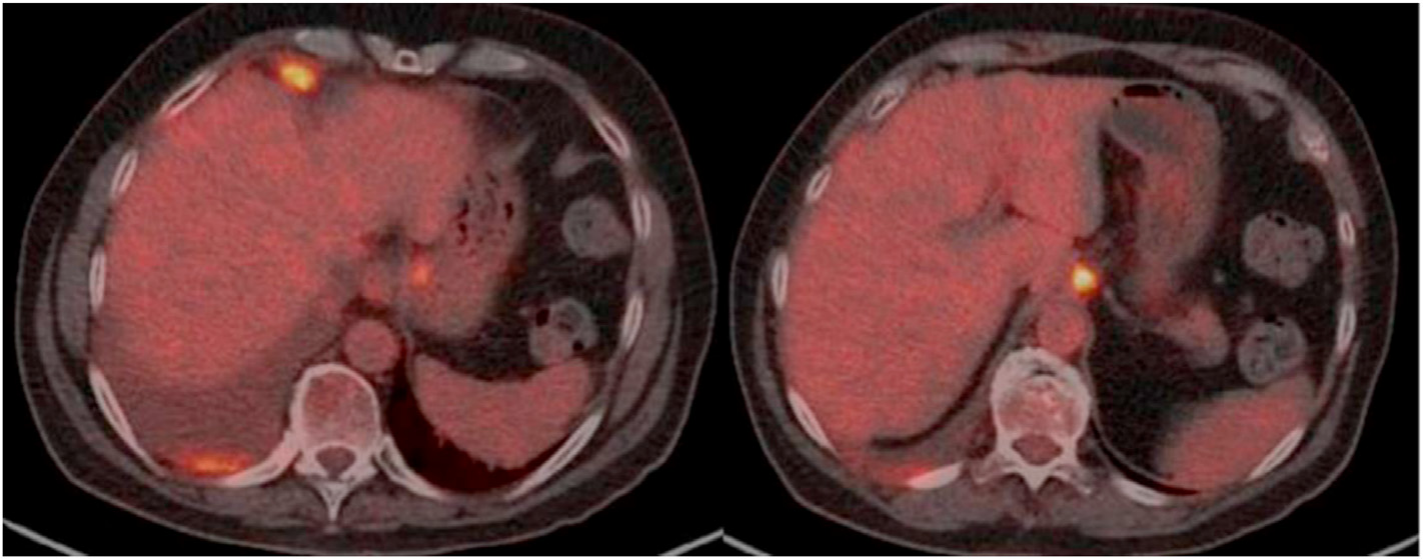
Case 2. PET/CT scan (September 28, 2021). ^18^FDG uptake in the apical, posterior and lateral right pleura with ipsilateral pleural effusion. Hypermetabolic new lesion in the right cardiophrenic space, and lymph nodes with high 18-FDG uptake in the area of the gastrohepatic ligament and pre-aortic.After confirming disease progression, initiating treatment with osimertinib at a daily dose of 80 mg was proposed. Initially, treatment tolerance was good. Unfortunately, the patient experienced clinical deterioration after three months of treatment, requiring hospitalization, and ultimately succumbed to disease progression.
